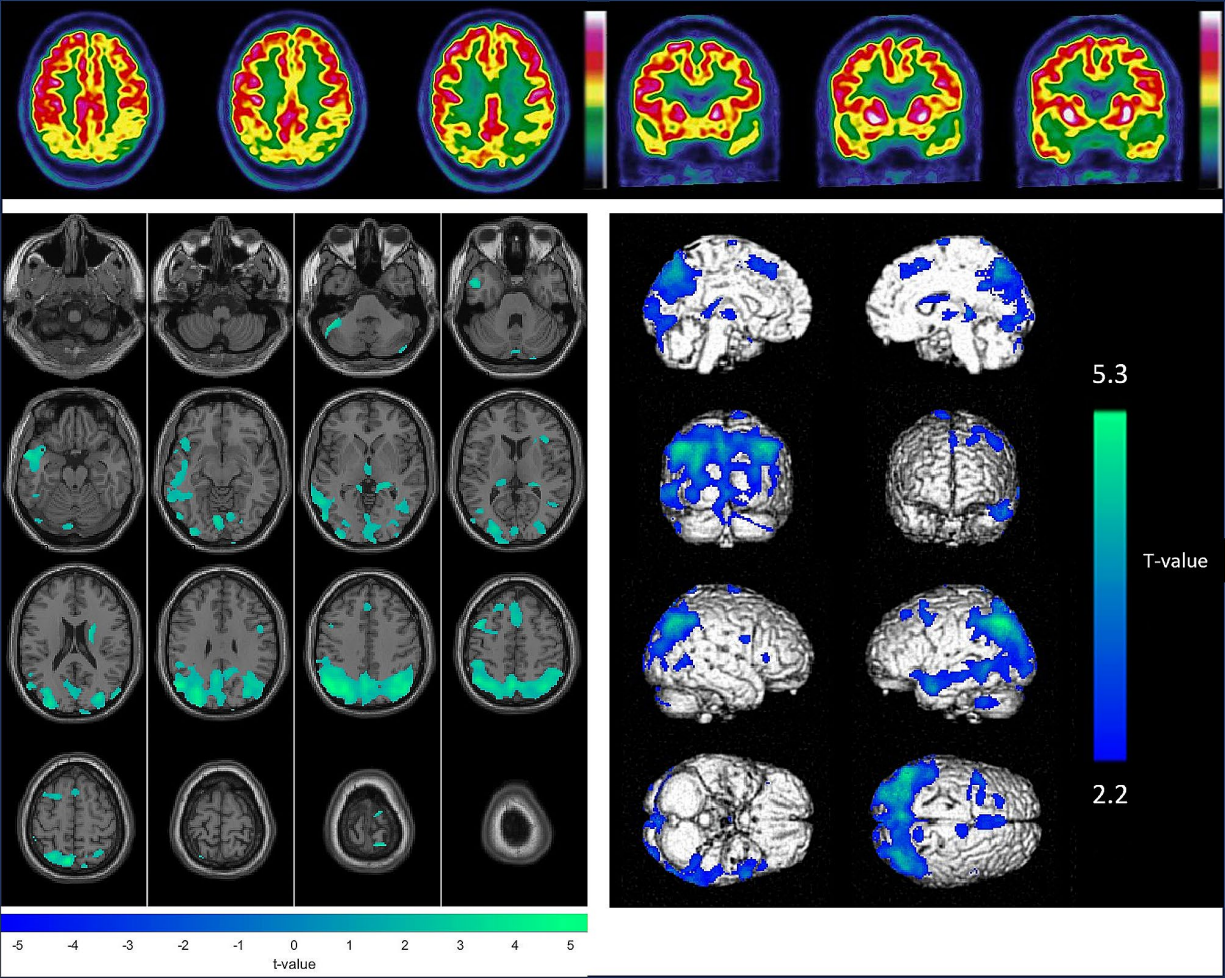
Example of the combined visual (upper panel, axial and coronal slices of brain ^18^F-FDG PET images) and semiquantitative (lower panel, with hypometabolisms in green projected on axial MR slices on the left and hypometabolisms in blue on a 3D volume rendered on the right) analysis for a 65-year-old male patient (initially diagnosed with MCI); these results were consistent with Alzheimer’s disease. The final diagnosis in the National Alzheimer’s database was Alzheimer’s disease and conversion to dementia after 31.5 months
